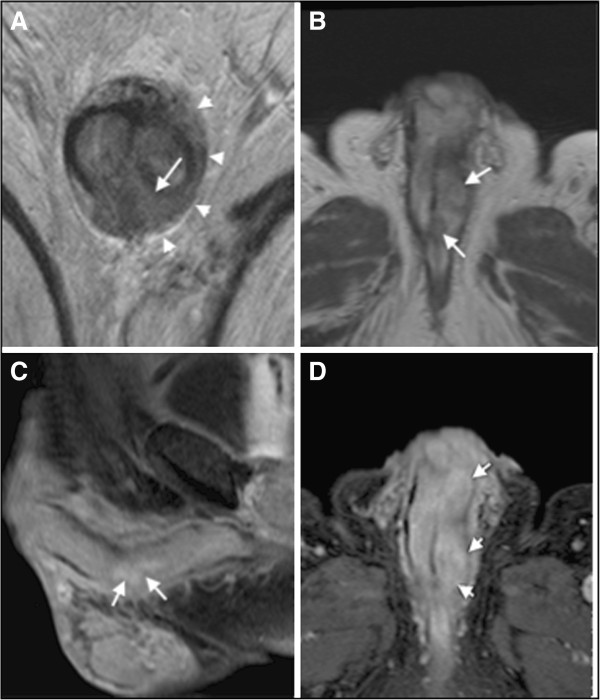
MRI of the penis. A) T2 weighted image shows a ipointense tissue sorrounding the left corpus spongiosum (arrow) and arising dorsal and ventral side of tunica albuginea; Buck’s fascia was also involved on the left side (arrowhead). B) Unenhanced axial T1-weighted MR image showing hypointense nodular lesions (arrows) on the left corpus spongiosum with irregular margins. C-D) Sagittal and axial T1-weighted MR images with fat signal suppression, after Gadolinum injection, show slight enhancement of the corrisponding tissue on A and B, as we expected on predominant fibrous matrix. None involvement of perivisceral fat was noted.
